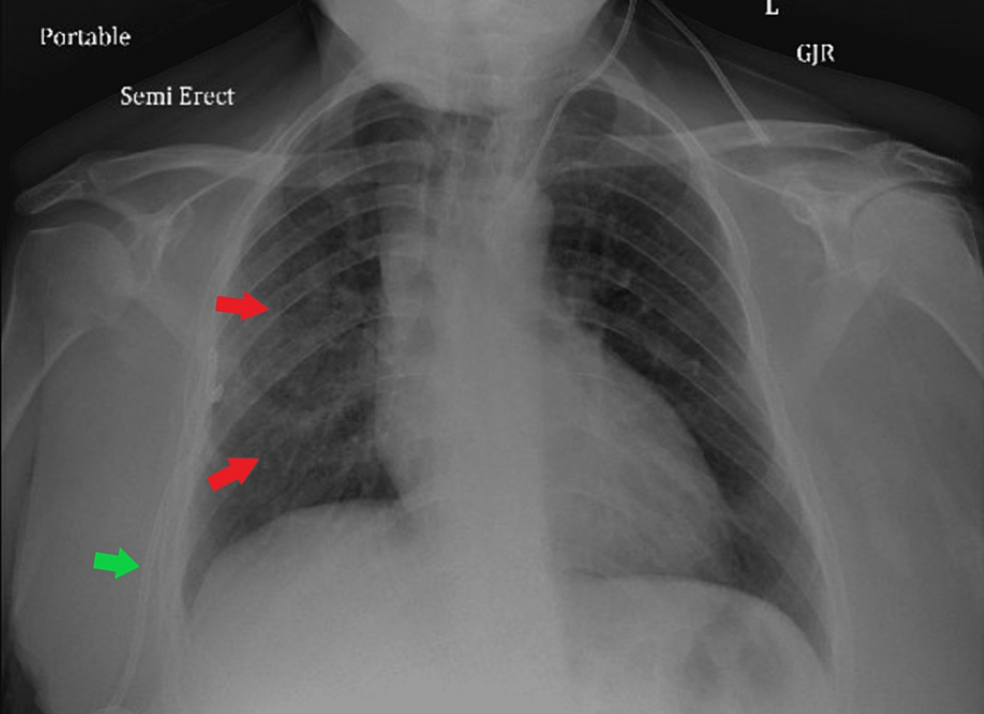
Follow-up chest X-ray revealing consistent opacities with a right-sided chest tube in place.
